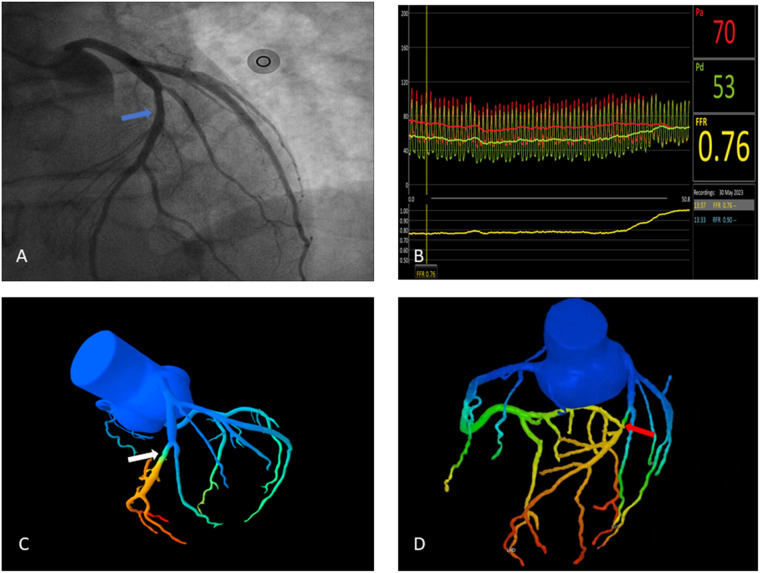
Representative case of a 53-year-old male with stable chest pain. (A) Invasive coronary angiography (ICA) showing a significant stenosis in the mid-left anterior descending artery (LAD; blue arrow). (B) Invasive FFR measurement confirming functional ischemia with a value of 0.76. (C) DL-based CT-FFR color-coded map showing a comparable result (CT-FFR = 0.75; white arrow). (D) CFD-based CT-FFR color-coded map demonstrating diagnostic consistency (CT-FFR = 0.74; red arrow).
